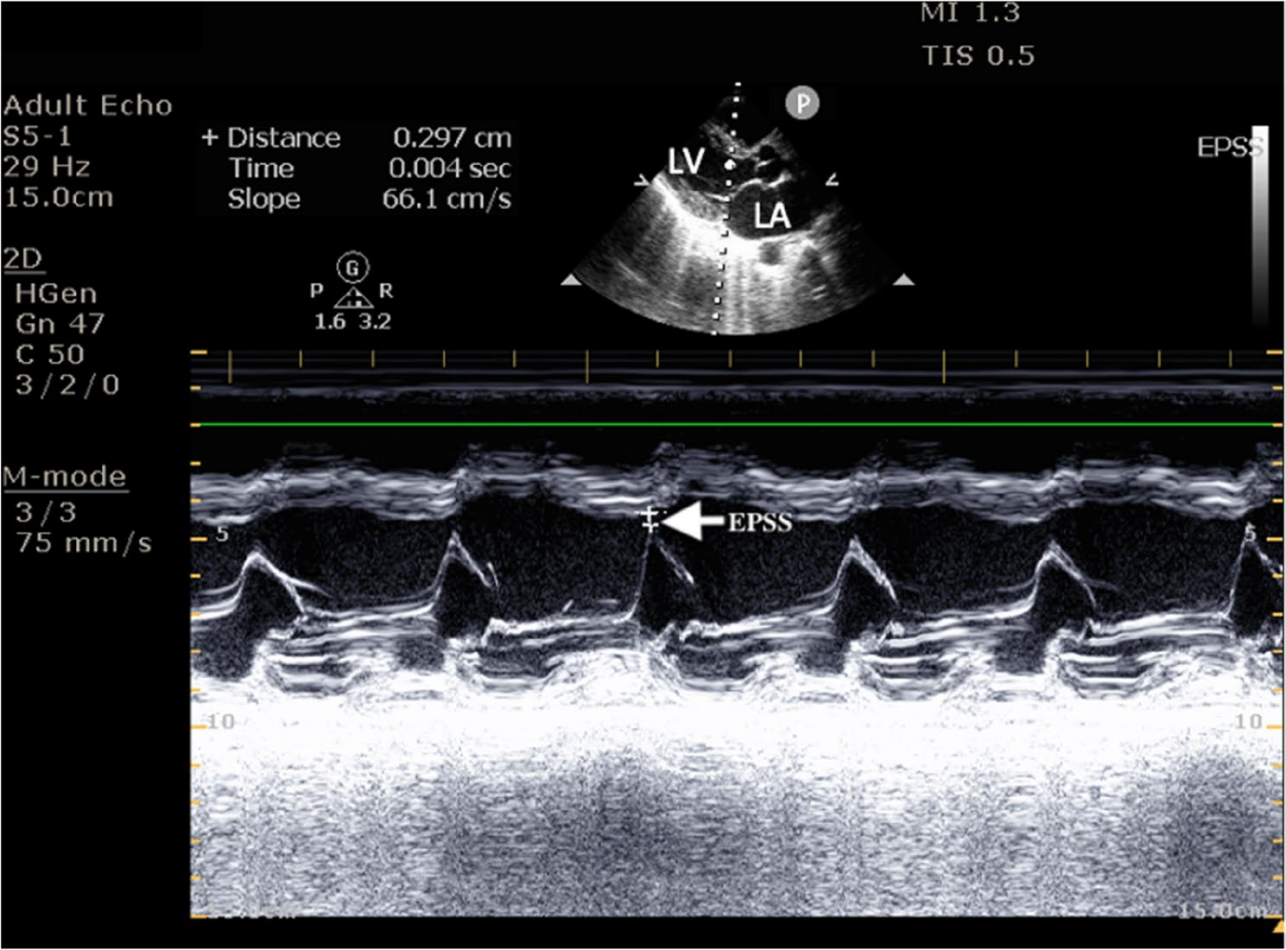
Echocardiographic Measurement of EPSS. Shows the echocardiographic view of EPSS measurement- 2.9 mm. EPSS = End Point Septal Separation, LV = Left Ventricle, LA- Left Atrium
